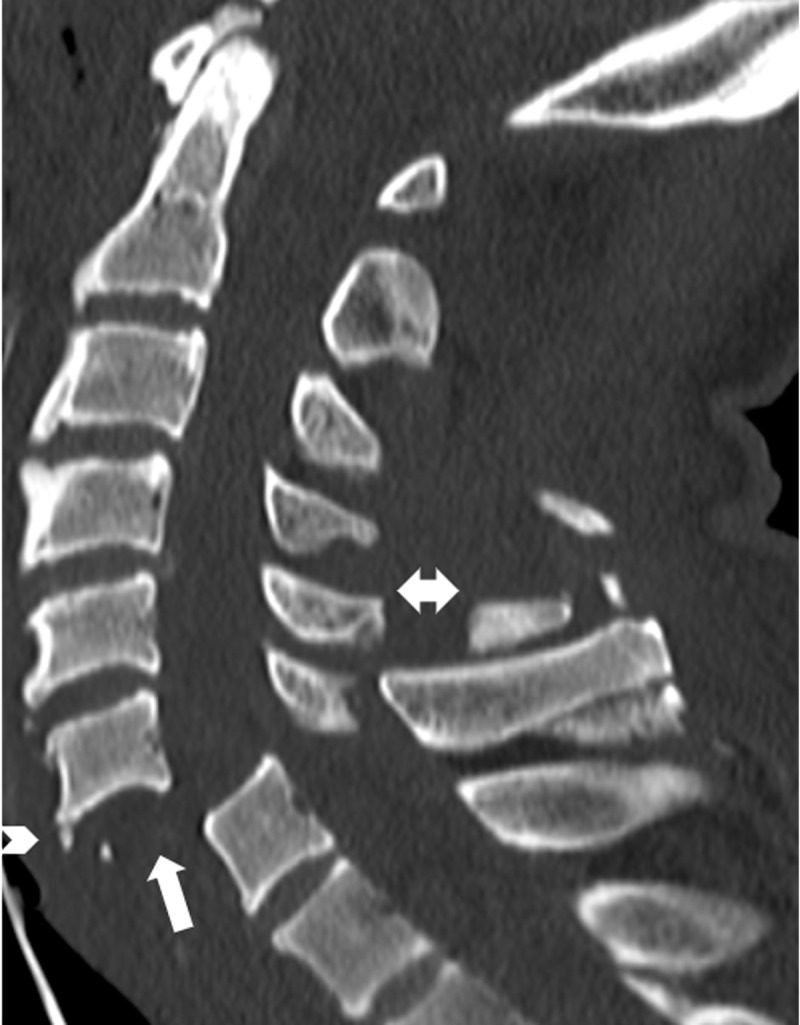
Floating vertebra sign and teardrop sign. Sagittal CT cervical spine of a 77-year-old man following a high energy motor vehicle accident shows 100% translation of the C6 on C7 vertebral bodies, which is consistent with a grade IV anterolisthesis. The appearance of a “floating vertebra” (upward pointing arrow) is associated with bilateral facet joint dislocation and injury to the posterior ligamentous complex. In addition, there are fractures involving the C5 and C6 spinous processes (double head arrow). The mechanism of injury is secondary to forceful muscle contraction, transmitted through the supraspinous ligaments. The tremendous contraction force produces the avulsion fractures of the spinous process known as clay-shoveler fracture. Note the presence of a tiny anterior “teardrop” fracture at the anterior and inferior aspect of the C6 vertebral body (arrow head).
